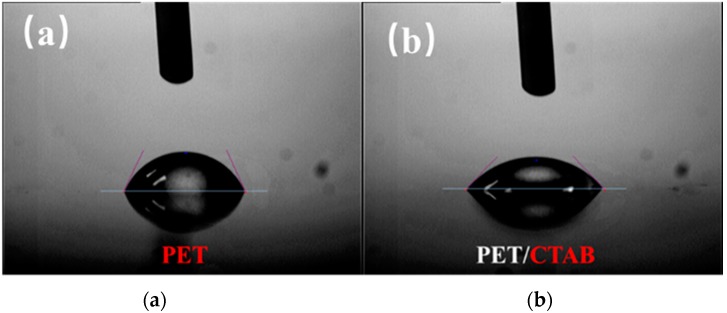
Contact angle of the surface of (a) neat PET and (b) PET/CTAB.
